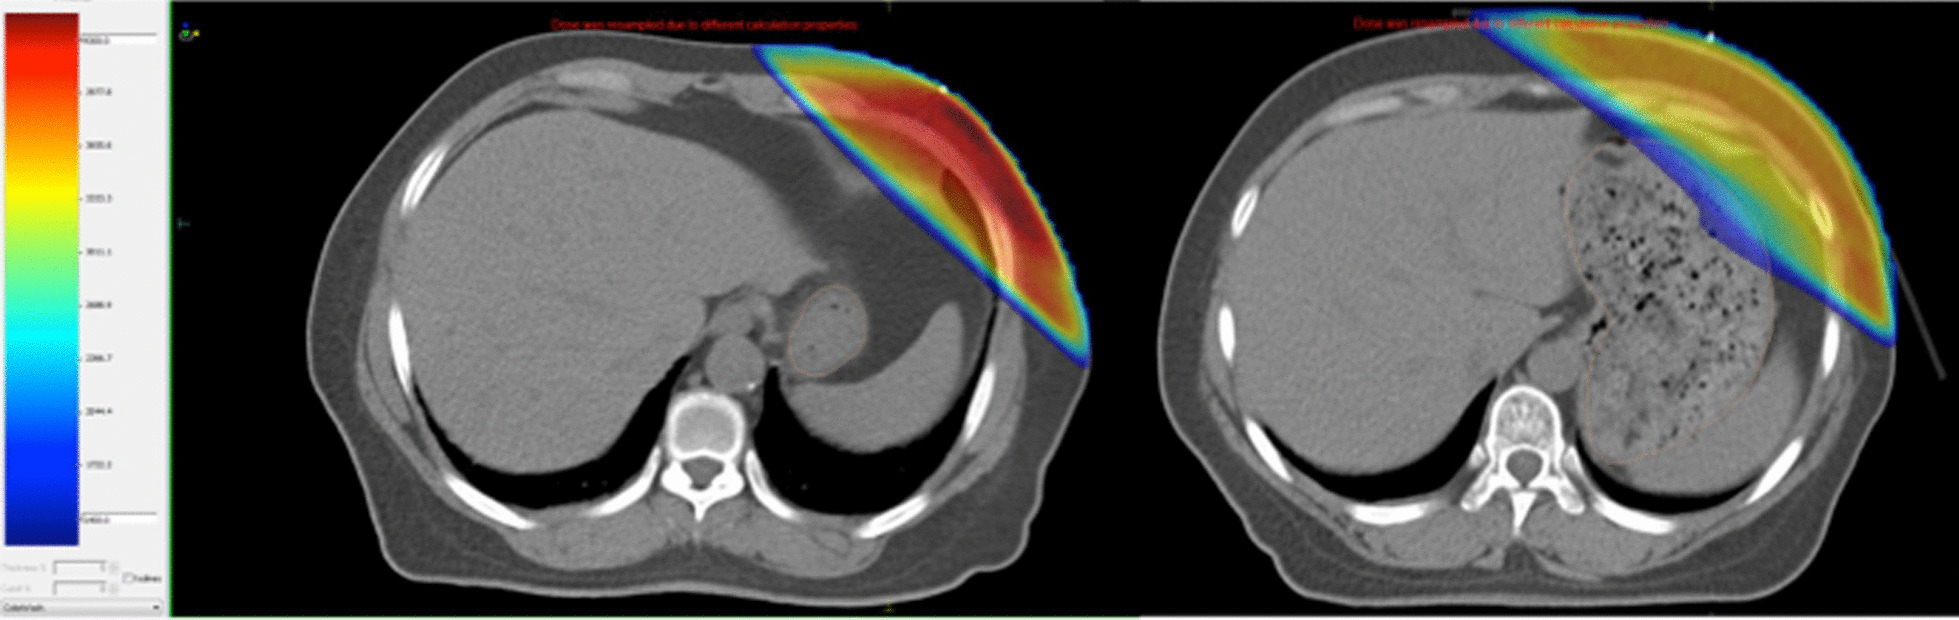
Computed tomography scans for 2 patients with stomachs in small size (left) and huge size (right). The dosimetric distributed in stomach quite different on account of different stomach volumes
